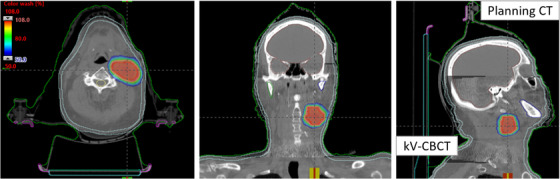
Overlay of the pre‐treatment kV‐CBCT with the planning CT images on the axial, coronal, and sagittal views of HyperArc VMAT SRT treatment of the same patient. In addition to anatomical landmarks, the planned dose colorwash (50%–108% isodose cloud) was overlaid at the treatment console for this treatment to demonstrate the delivery accuracy. The CBCT images were acquired in the treatment position and rigid‐registration was performed via automatic image‐registration with well‐defined region of interest followed by manually fine‐tuning the registration for better alignment of the target and spinal cord via soft‐tissue matching
